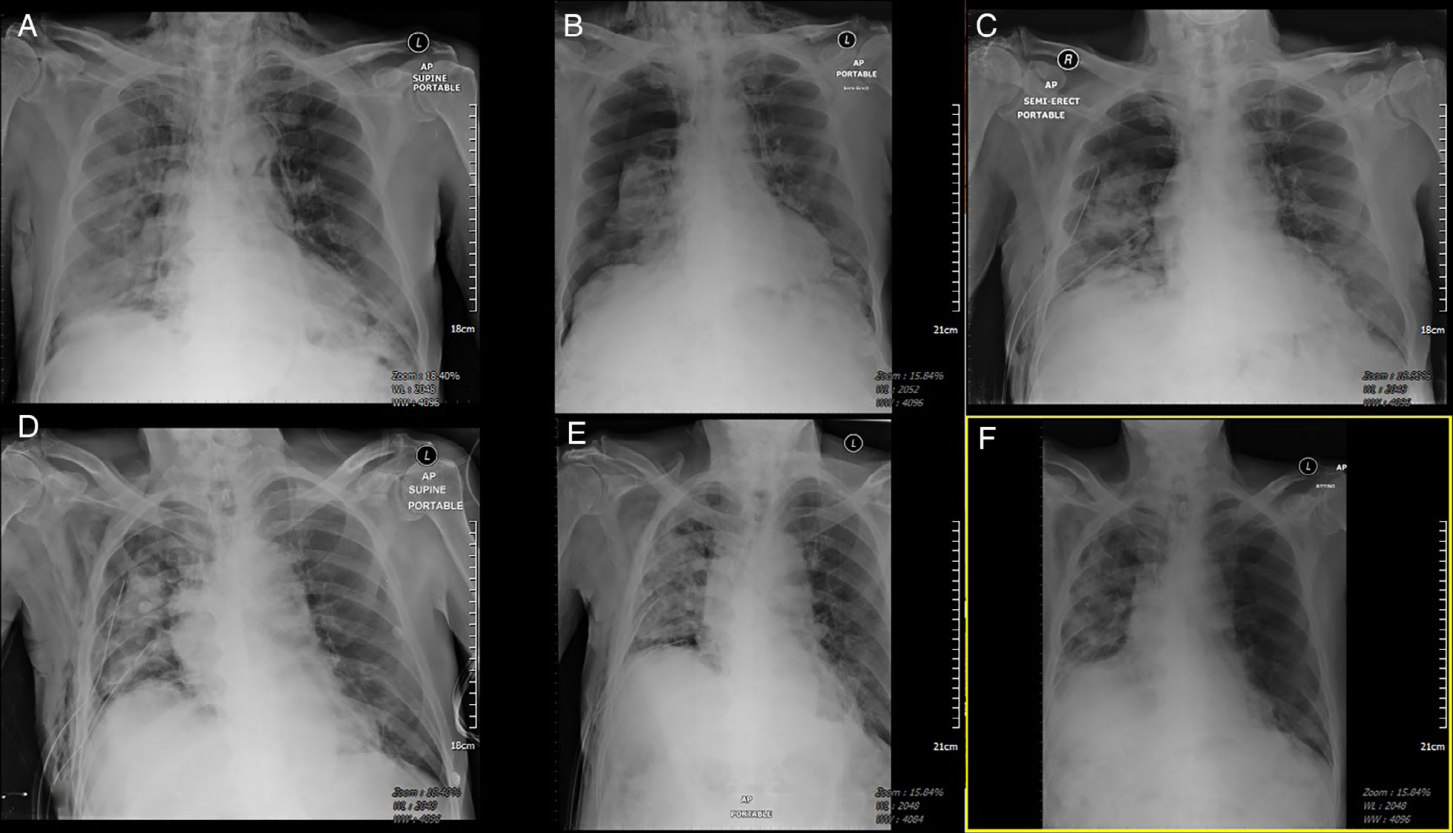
Serial chest radiograph. (A) Day 13 of illness with rim of air surrounding cardiac border (pneumopericardium) and air seen in subcutaneous tissue (subcutaneous emphysema [SCE]). (B) Day 15 of illness with large right pneumothorax (PTX). (C) Day 20 of illness with persistent air leak (5 days) and poor lung expansion. (D) Day 24 of illness with persistent air leak (9 days) and poor lung expansion with worsening SCE. (E) Day 26 of illness with cessation of prolonged air leak and lung fully re‐expanded after two doses of autologous blood pleurodesis. (F) Day 29 of illness with resolution of PTX and no recurrence after 24 h of removal of intercostal chest drain
